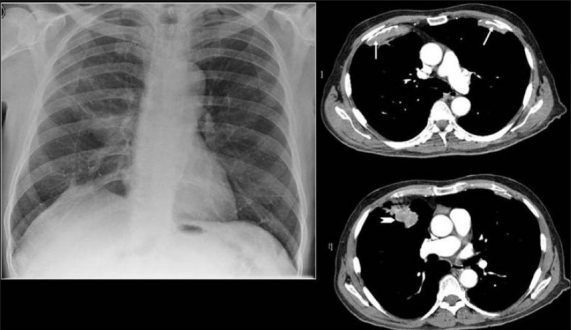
A conventional radiograph of patient presenting with hemoptysis and weight loss shows a right hilar mass but no calcification is apparent. Two sections of axial CT scans show calcified pleural plaques (white arrows) due to previous asbestos exposure complicated by a bronchogenic neoplasm (arrow head)
